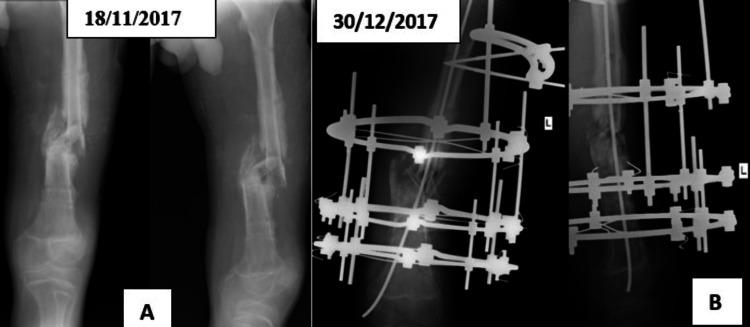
(A) Anteroposterior and lateral X-rays show a non-united fracture. (B) X-ray after fixation with ilizarov ring fixator as well as TENS nail in situ. TENS: Titanium Elastic Nailing System.
